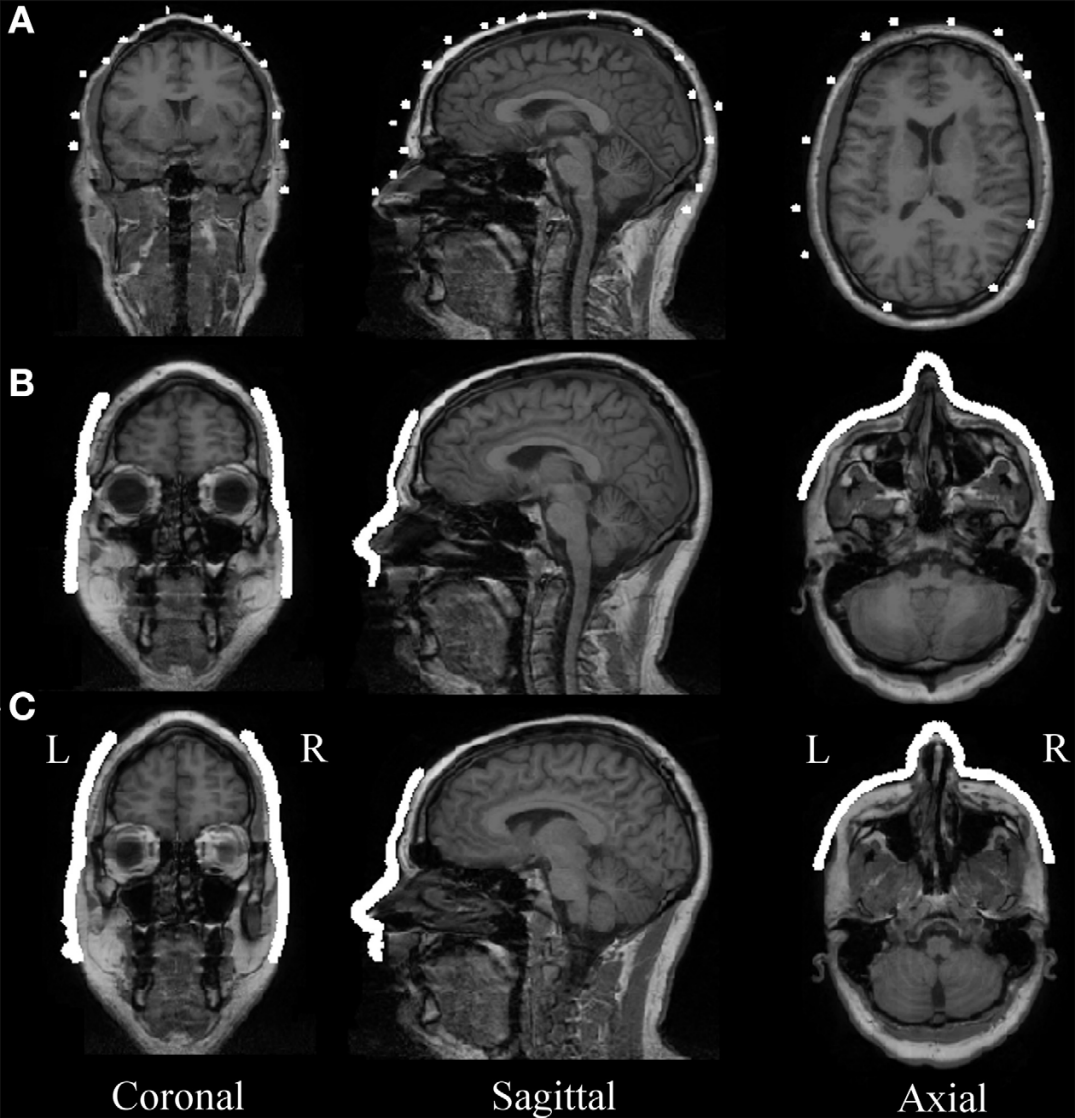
MEG-MRI Co-registration. (A) Polhemus, (B) laser scanner, and (C) Kinect scanner head digitization data are overlaid on the MR image for a representative participant, after automatic co-registration. Different slice is used for Polhemus to highlight co-registration accuracy in slices that included the most digitization points.
